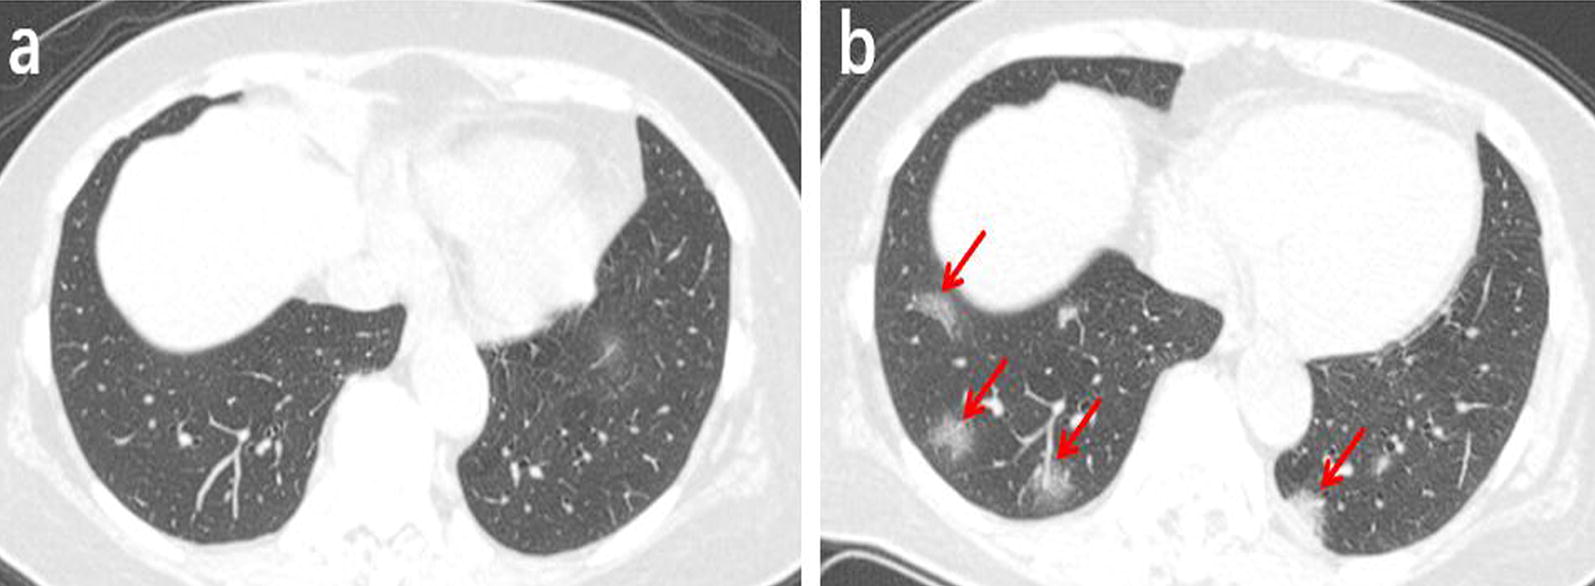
A 64-year-old female Wuhan resident presented with fever and coughs for 3 days. a The first axial-view chest CT scan shows no lesions. b The second axial-view chest CT scan conducted 4 days after the first CT shows the presence of multiple ground-glass opacities in the lower lobe, located below the pleura with clear margins. Thickened vascular are visible inside the lesions in the lower right lobe (red arrows)
